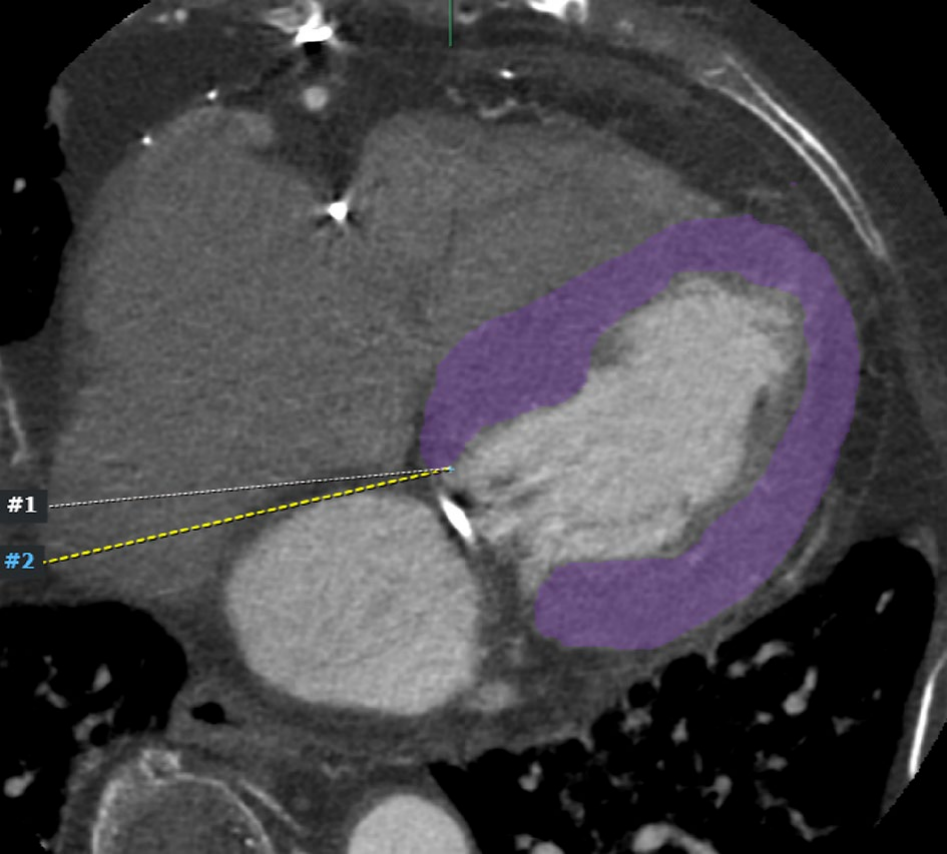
Axial cardiac CT image of a 77-year-old female patient. The myocardium (purple color) is segmented by excluding the LV blood pool and trabeculae to improve reproducibility for delineating the endocardial border. CT, computed tomography; LV, left ventricular.
